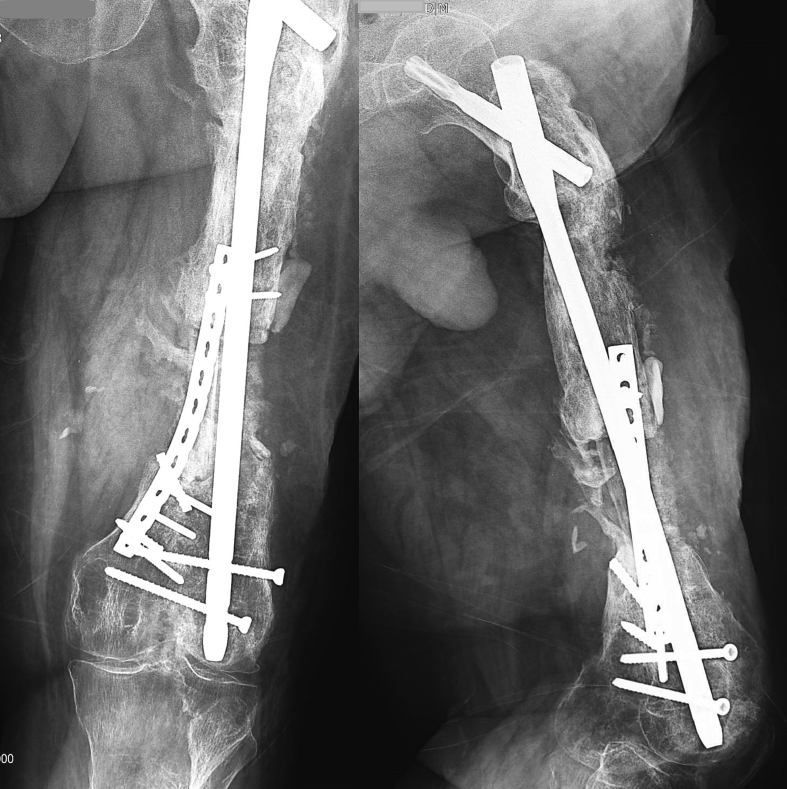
Patient's radiograph 9 months after free fibula bone graft from medial approach.
